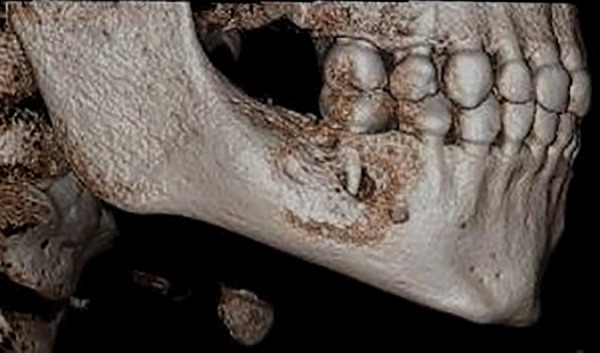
Computed tomography scan of right lower area. Note: Extensive bone resorption of the buccal cortical plate of tooth 85 with the MB root being in contact with the oral soft tissues
